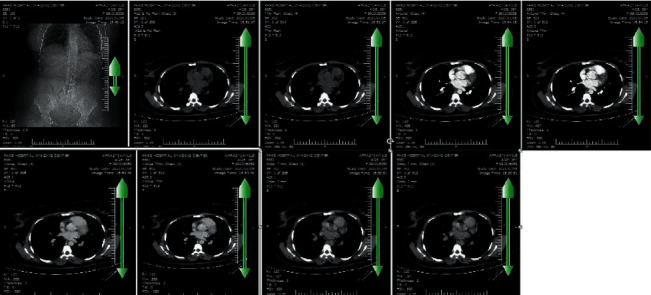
Multiple images of the patient's CT scan.
